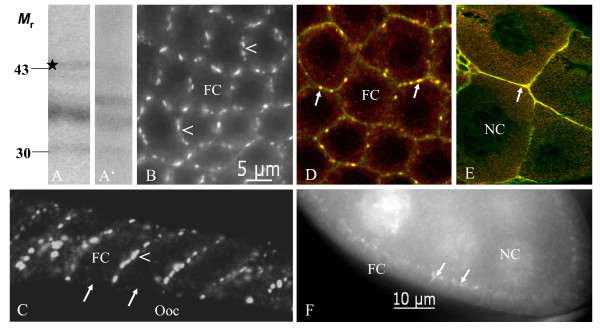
Inx3 is colocalized with Inx2 to membrane plaques in follicle cells and nurse cells. On immunoblots of ovary extracts using Inx3-antisera (A: AInx3GP-CT, A': AInx3Rb-CL) a weak band at the calculated molecular mass of 45 kDa (asterisk) and two bands at 35 and 30 kDa are recognized. B, C: Inx3 is localized to presumed gap-junction plaques between follicle cells (stage 10a). It is expressed in a continuous as well as punctate pattern at the lateral membranes (arrowheads, B: AInx3Rb-CL, WFF, C: AInx3GP-CT, LSM), but is missing at the apical membranes and at the oolemma (arrows). D, E: Inx2 (red, AInx2Rb-CT(REM)) and Inx3 (green, AInx3GP-CT) are colocalized in lateral follicle-cell membranes (yellow, arrows in D, stage 10a, LSM) as well as in nurse-cell membranes (yellow, arrow in E, stage 10b, LSM). F: In young follicles, Inx3 is located in plaques between nurse cells and follicle cells (arrows) and around nurse-cell nuclei (stage 7, AInx3Rb-CL, WFF). For further abbreviations, see legend to Fig. 1.
